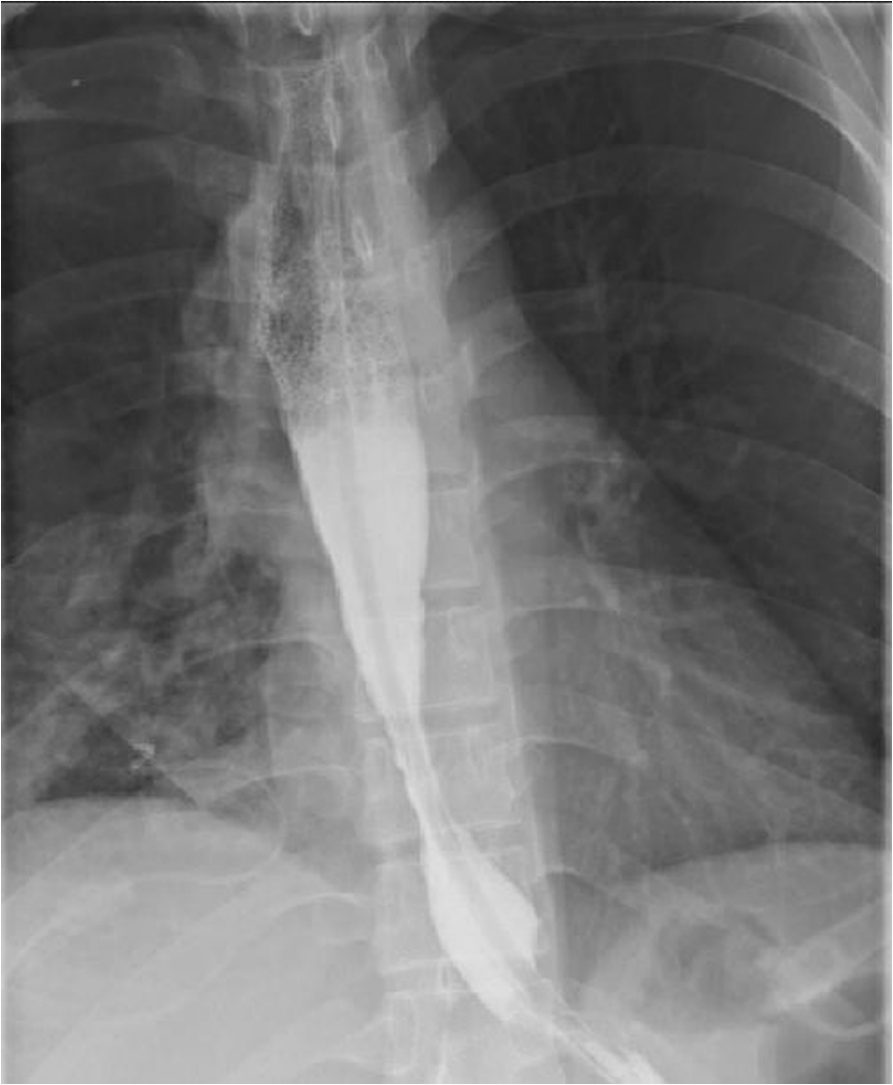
Postoperative contrast radiography of esophagus before recovery to normal diet.
